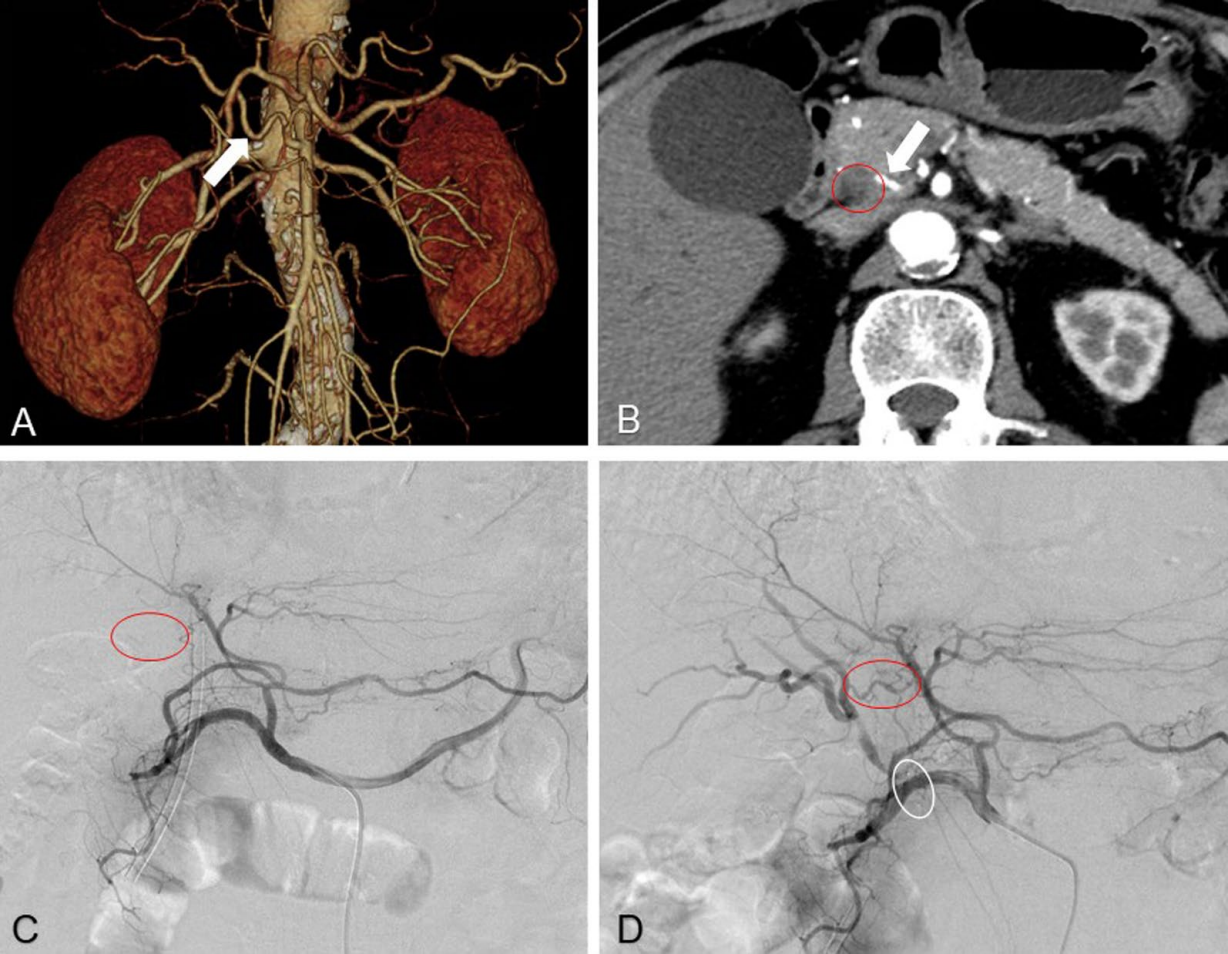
Case 4: Imaging findings of a representative case of preoperative embolization of rRHA. A rRHA arising from SMA by CT angiography (white arrow). B CT scan showed the main tumor of pancreatic head cancer (red circle) had closely contact with rRHA, which ran into the parenchyma of the pancreas (white arrow). C Before embolization of the rRHA, the vascular supply to the right lobe from the LHA was scanty (red circle). D After embolization of the rRHA by the plug (white circle), blood flow of right lobe of the liver was supplied via the communicating arcade from LHA, which went through the hilar plate (red circle). *rRHA* replaced right hepatic artery; *SMA* superior mesenteric artery; *CT* computed tomography; *LHA* left hepatic artery
